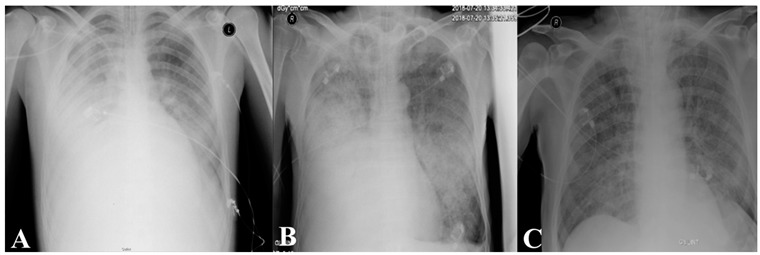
Figure 1. Final X-rays of the patients: A—1st case; B—2nd case; C—3rd case.
